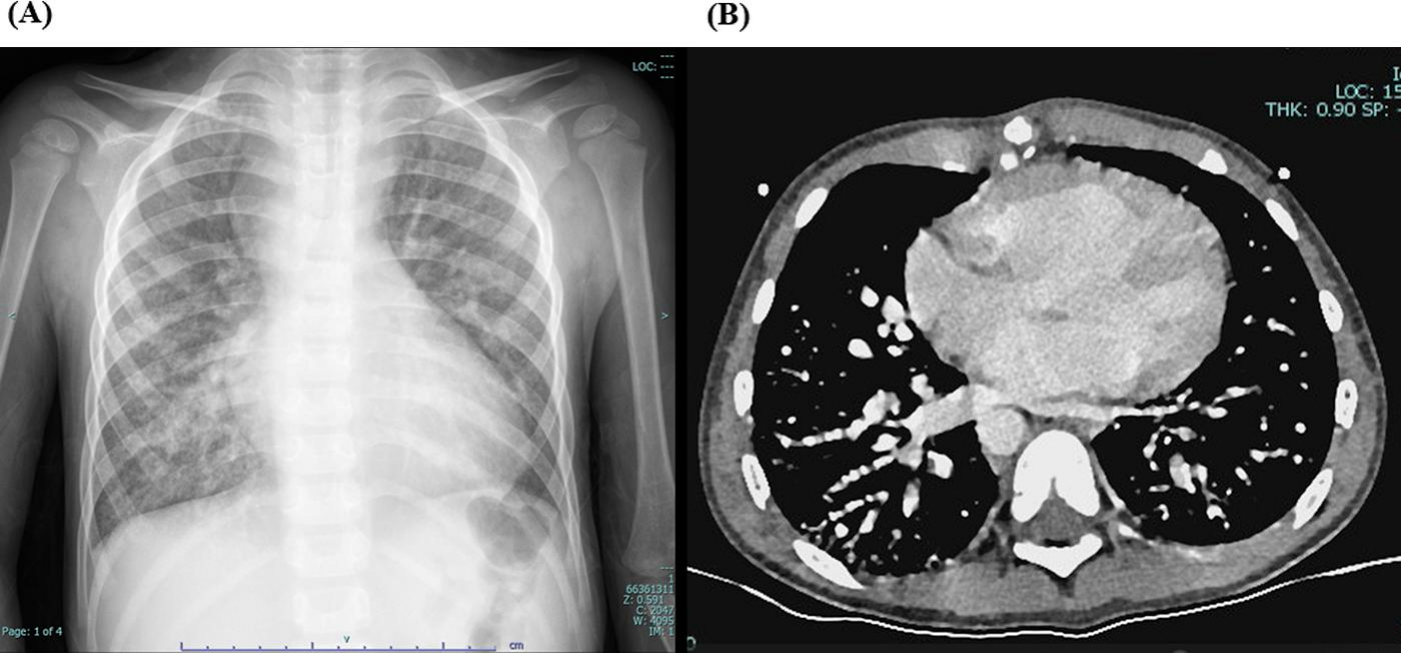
(A) preoperative chest X-ray showing increased pulmonary markings. (B) Preoperative contrast-enhanced cardiac CT demonstrating simultaneous opacification of pulmonary arteries and veins, with a beaded appearance of the distal pulmonary arteries, more prominent in both lower lobes, consistent with diffuse pulmonary arteriovenous malformations (PAVMs).
